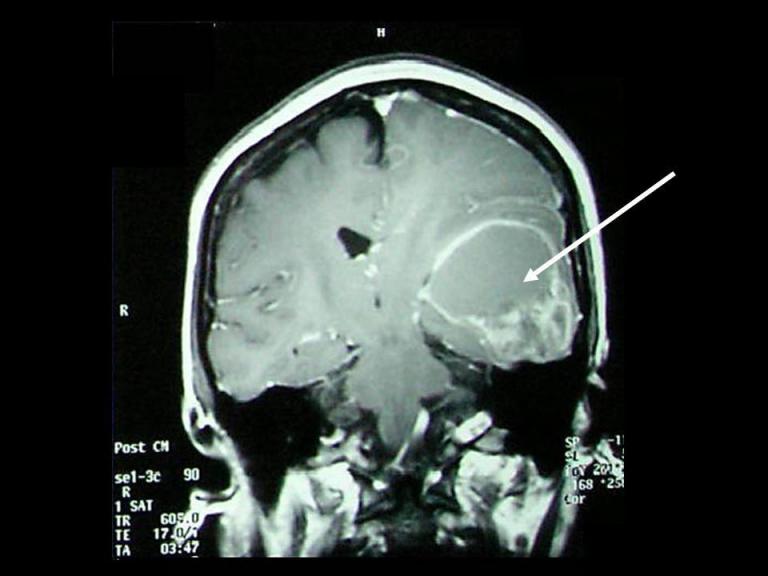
A coronal contrast-enhanced MRI of the brain demonstrating the heterogeneously enhancing left temporal lobe mass (glioblastoma multiforme) with an associated cystic component (arrow).
